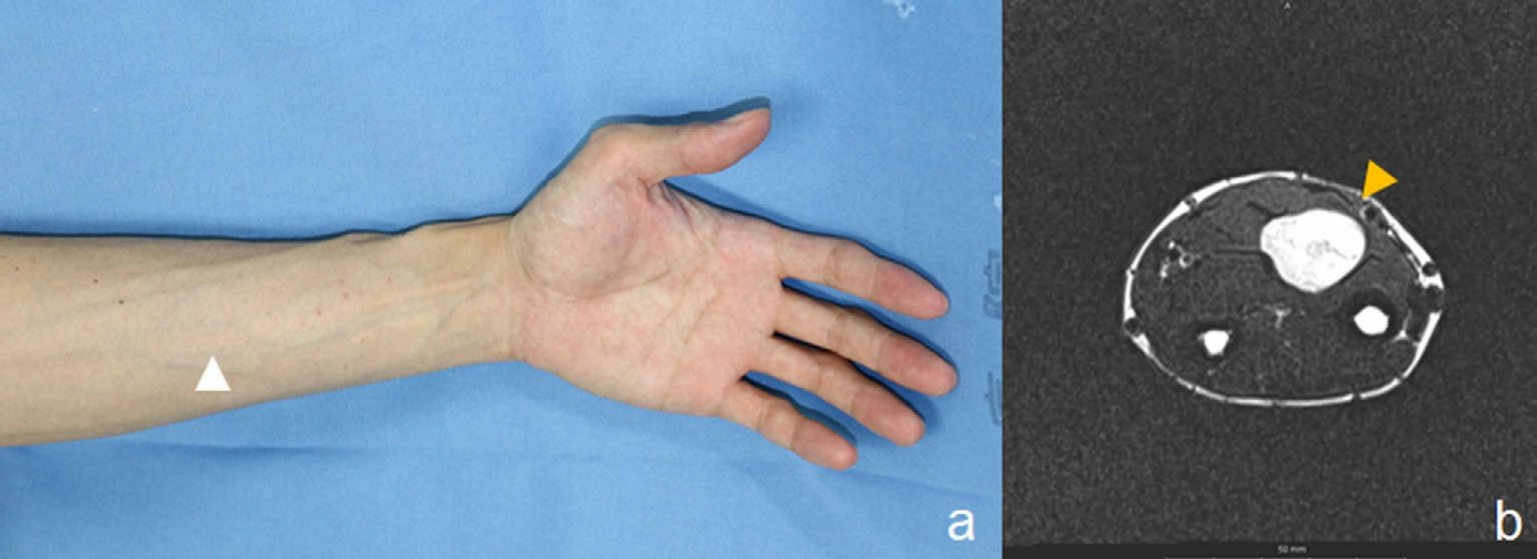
(a) The schwannoma on the flexor side of the forearm. (b) The magnetic resonance image shows that the schwannoma involves the median nerve in the forearm (arrowhead).
